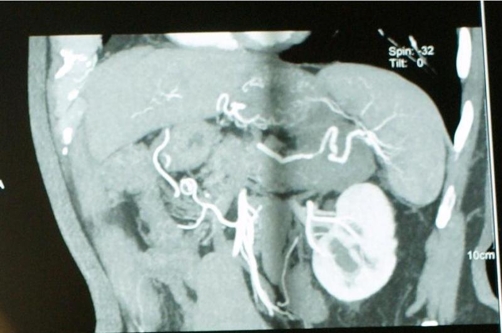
Computed tomography-angiography showing that the tumor distorted and compressed the splenic vessels.
